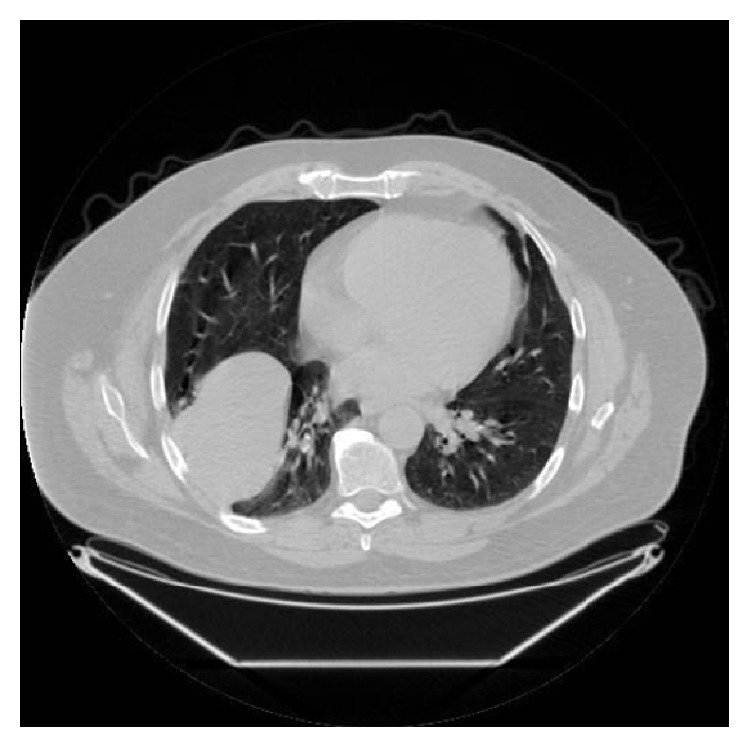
Chest computed tomography scan in lung window shows a well-circumscribed large solid mass.
